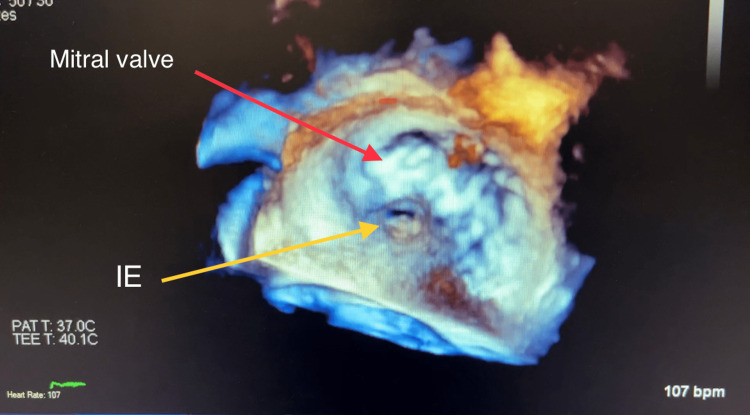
Three-Dimensional Transesophageal Echocardiogram Image Showing Infective Endocarditis of Mitral Valve With Anterior Leaflet Perforation
